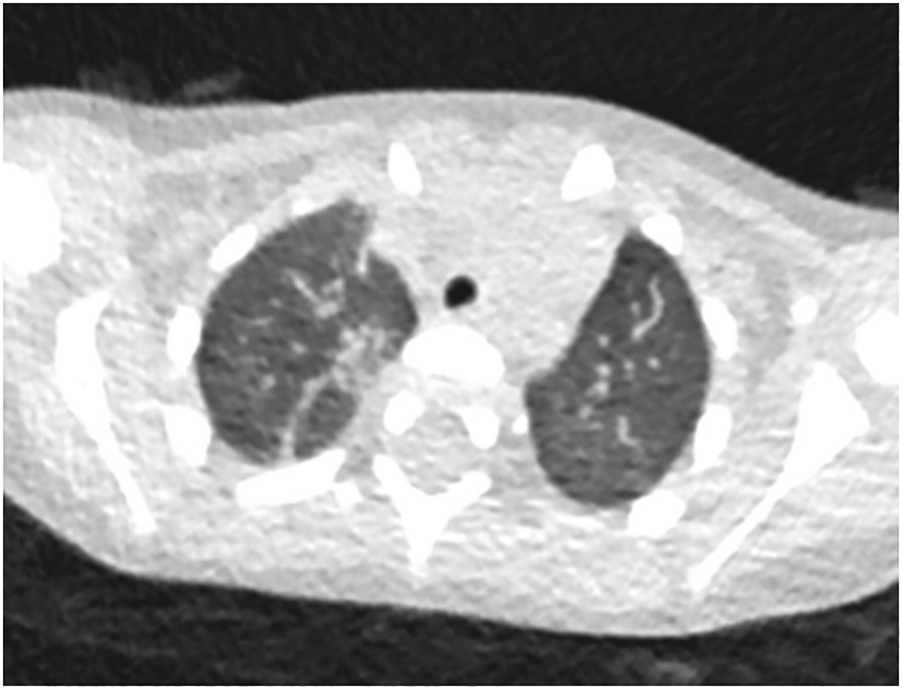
22-month-old boy infected with the Omicron variant of SARS-CoV-2. Unenhanced chest CT scans show consolidation in the apical segment of the right upper lobe. CT findings were as-signed CT severity score of 1.
